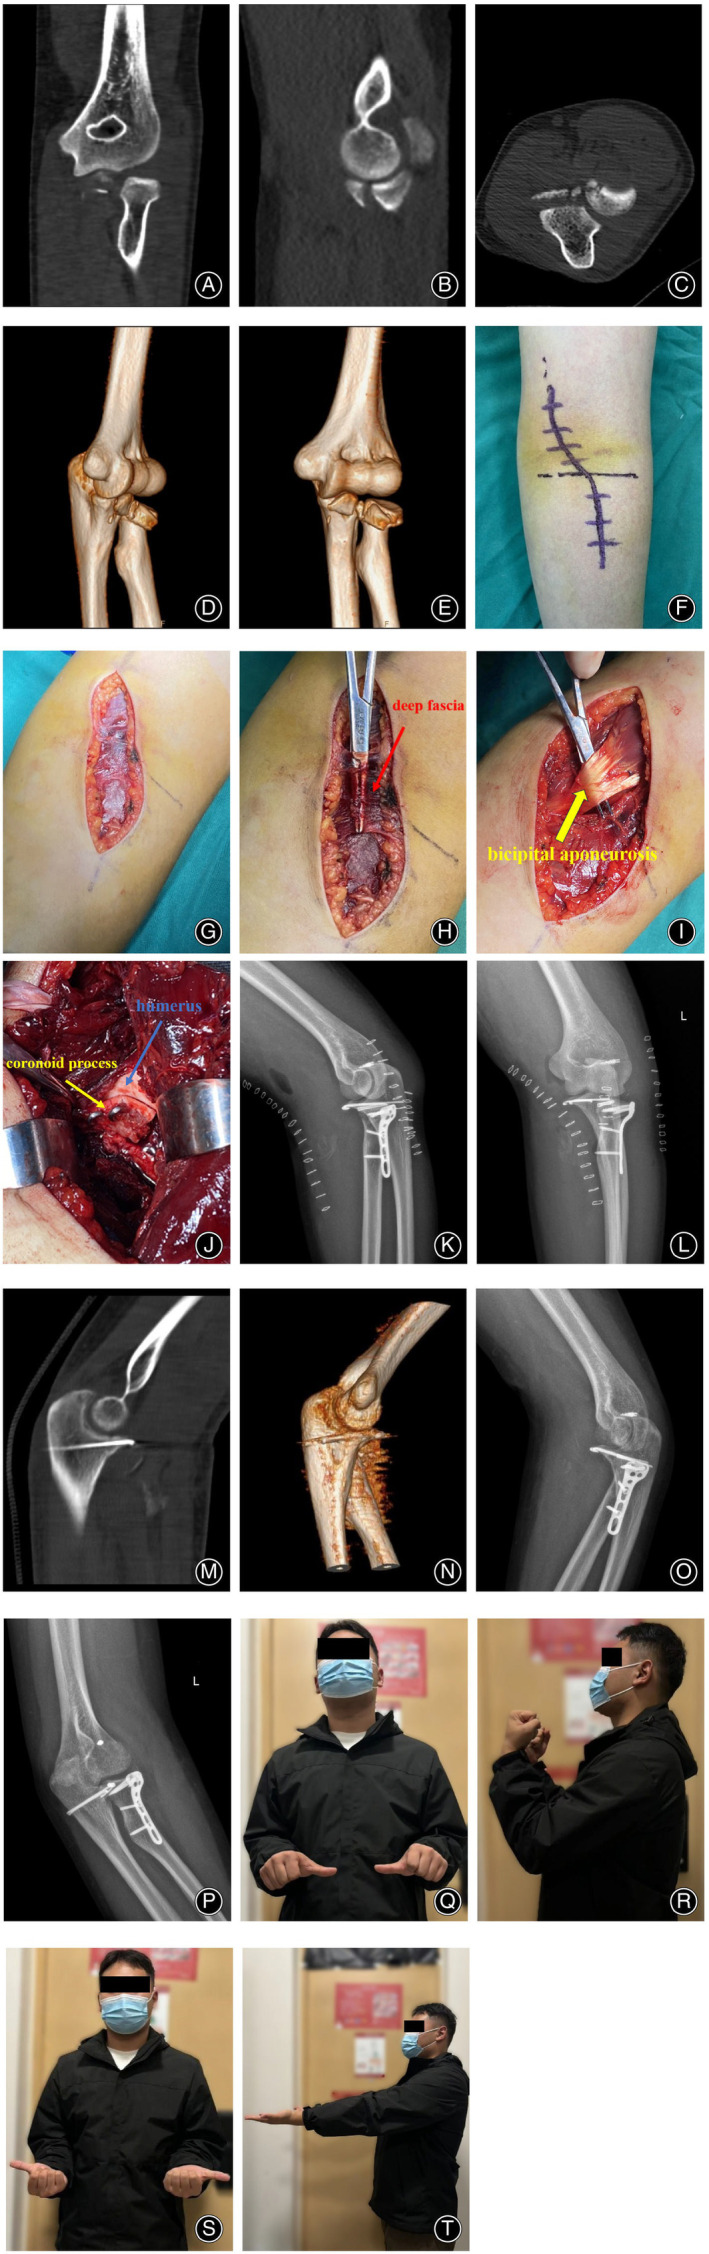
Typical case 1. A 21‐year‐old man suffered from sports‐related injuries and received treatment with k‐wires. (A–E) Preoperative CT images showed ulnar coronoid process fracture and radial head fracture. (F–J) Positioning of the surgical incision and exposure of surgical area. (K–N) Postoperative x‐ray and CT images showed an anatomical reduction of the coronoid process fracture and radial head fracture. (O, P) Ten weeks after the operation, the radiographs of the elbow joint showed that the fracture was completely healed. (Q–T) The function of the patient's elbow joint at the last follow‐up was favorable, and the patient was pain free.
